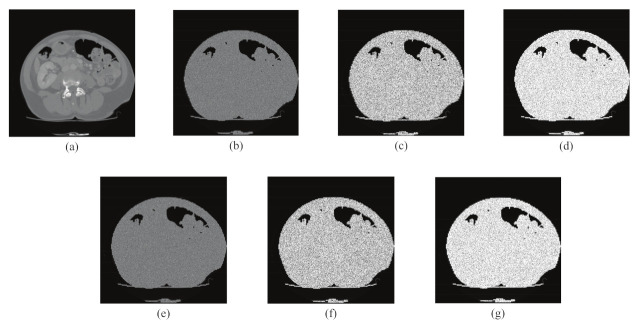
Key sensitivity test for CT Abdomen in decryption processes. (a) Decrypted image with correct KEY; (b) decrypted image with key KEY1; (c) decrypted image with KEY2; (d) decrypted image with key KEY3; (e) decrypted image with key KEY4; (f) decrypted image with key KEY5; and (g) decrypted image with key KEY6.
